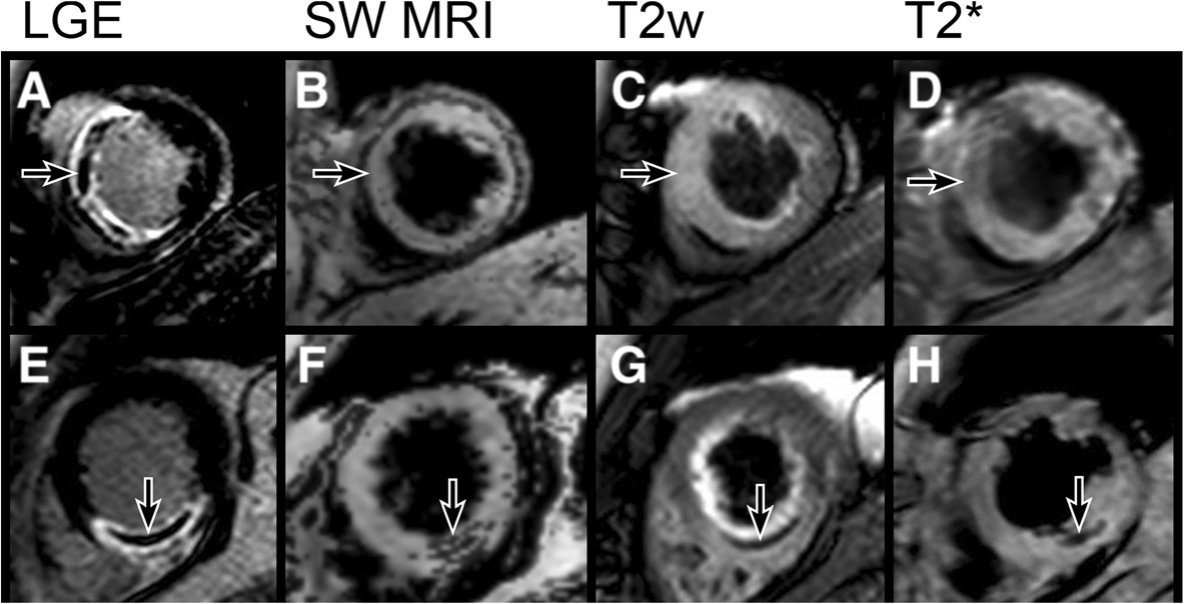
SW MRI comparison with T2w and T2*. Top row: MO as shown by LGE imaging (A, arrowed) does not correspond to hypointense myocardium indicating absence of IMH on SW MRI (B), T2w (C) and T2* (D) images. Bottom row: another patient has MO visible on LGE (E, arrowed) corresponding to hypointensity on SW MRI (F, arrowed), T2w (G, arrowed) and T2* (H, arrowed) images indicating presence of IMH.
